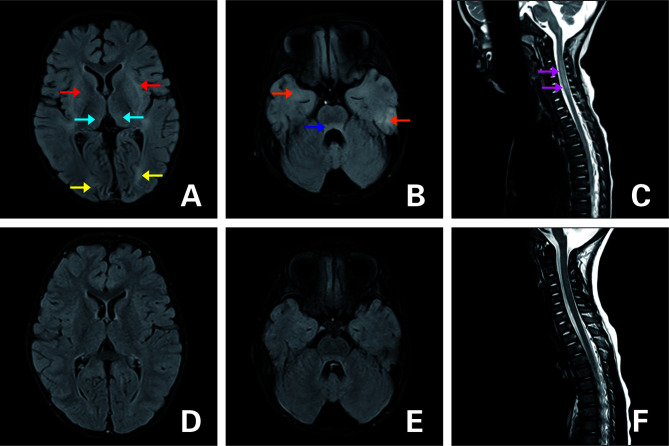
FLAIR MRI of the brain (A–C) upon admission and (D–F) 3 months later. (A, B) T2-hyperintense lesions in the dorsal thalamus (blue arrow), basal ganglia (red arrow), bilateral cerebral hemispheres (yellow arrow:occipital lobe;orange arrow:temporal lobe), and pontine (dark blue arrow). (C) Lesions in the cervical spinal cord (purple arrow). (D–F) Follow-up images of T2 lesions after they disappeared.
